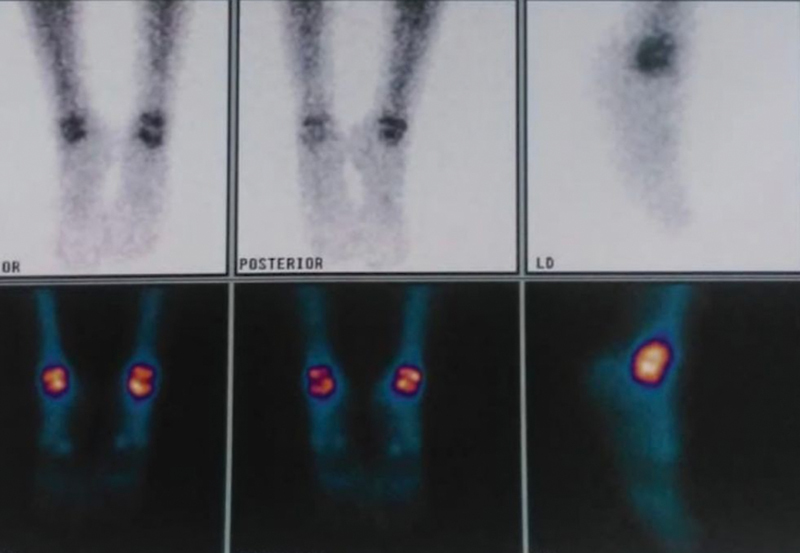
Ankle bone scintigraphy. Source: Service collection.
